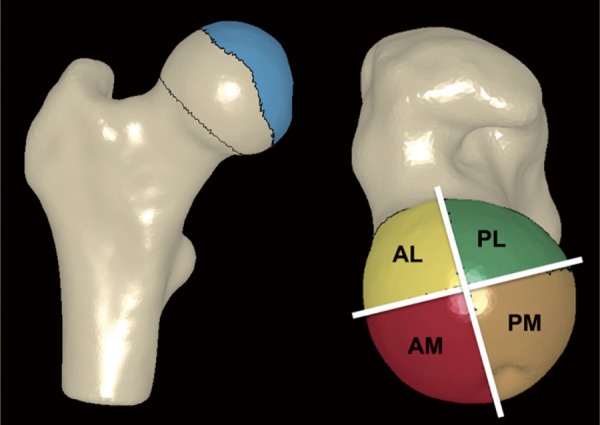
3D reconstruction of femur from CT image data from a control subject. Left: The femur head-neck junction was defined automatically (line at head-neck junction). The region of femoral head that was covered (blue) was determined by projecting the rim of the acetabulum to the femur (line representing boundary of covered region in blue). Right: Two planes were created at the center of the femoral head (white) to divide the head into four anatomical regions. A = anterior, P = posterior, M = medial, and L = lateral. Each region includes the portion of the head from the most superior aspect to the femoral head/neck junction inferiorly.
